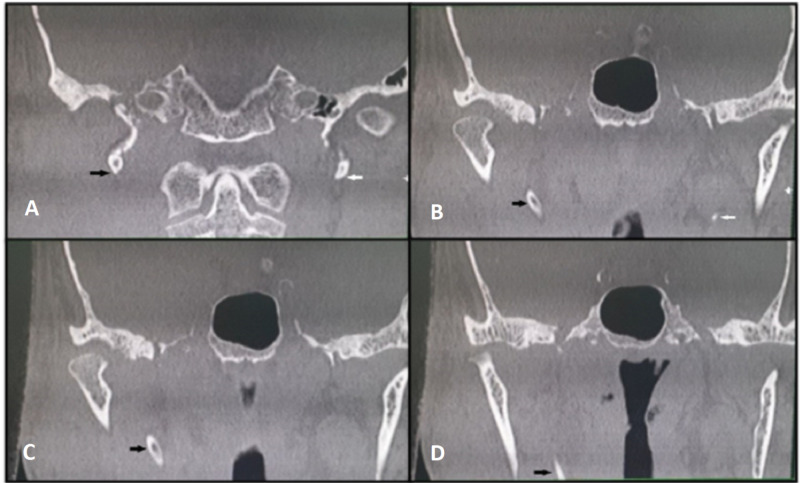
Serial coronal sections of CT scan of the head and neck Images (A-D) showing an elongated styloid process on the right side depicted by the black arrow. Note the normal styloid process on the left side (A and B) depicted by the white arrow.
